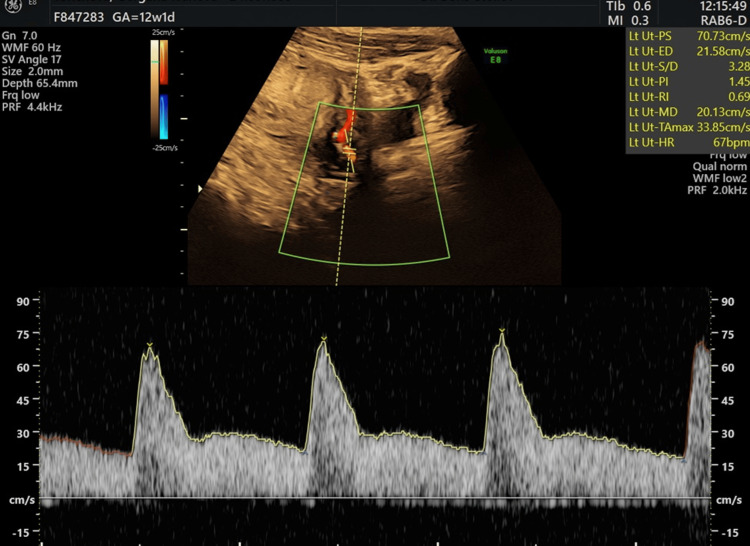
Doppler study: pulsatility index (PI) uterine artery (original image from the study)
